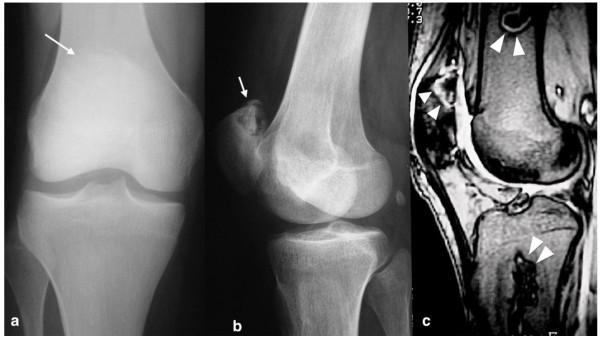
A 33-year-old patient with history of S.L.E. and hip ON, presented with a painful knee. The plain x-rays (a,b) revealed an ostenecrosis lesion at the superior pole of the patella (arrows). The sagittal fat suppressed T2-w TSE MRI (c) showed this ON lesion at the patella and two additional lesions at femur and tibia (arrowheads).
